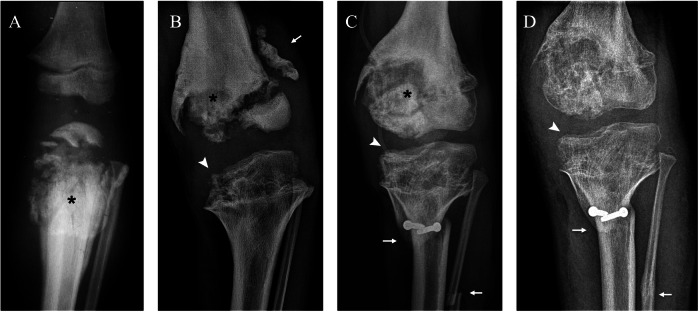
Neuropathic arthropathy of the left knee of a boy with hereditary sensory and autonomic neuropathy type IV. Anteroposterior radiographs at 3 (A), 5 (B) and 7 (C, D) years of age. Radiograph at injury (A) shows fragmentation of the tibial proximal metaphysis (asterisk). Radiograph (B) shows collapse of the tibial medial plate (arrowhead), lytic changes and fragmentation of the distal femoral epiphysis and metaphysis (asterisk) and bone fragments (arrow). Radiograph after oblique proximal tibial osteotomy (arrows) (C) shows partial healing of the tibial plate (arrowhead) and sclerosis of the femoral epiphysis (asterisk). Radiograph 2 months after surgery (D) shows progressive healing of the osteotomies (arrows) and the tibial plate (arrowhead)
